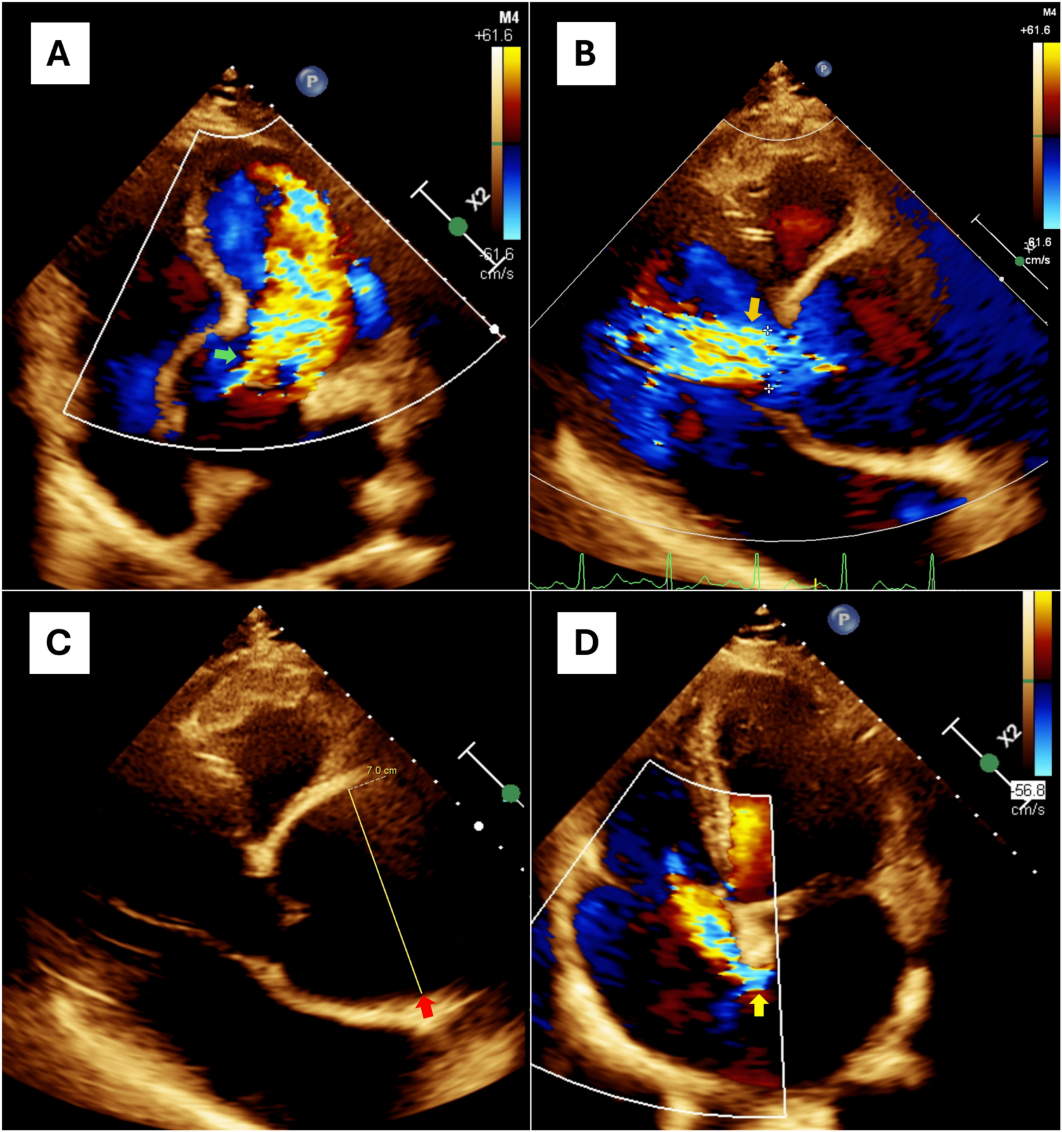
Transthoracic echocardiography findings A. Apical five-chamber view showing a severe aortic insufficiency jet (green arrow); B. Parasternal long-axis view demonstrating a holodiastolic aortic insufficiency jet (orange arrow); C. Aneurysmal dilation of the aortic root and loss of the sinotubular junction (red arrow); D. Apical four-chamber view, color Doppler imaging confirming a non-restrictive left-to-right shunt across the 11 mm atrial septal defect (yellow arrow).
